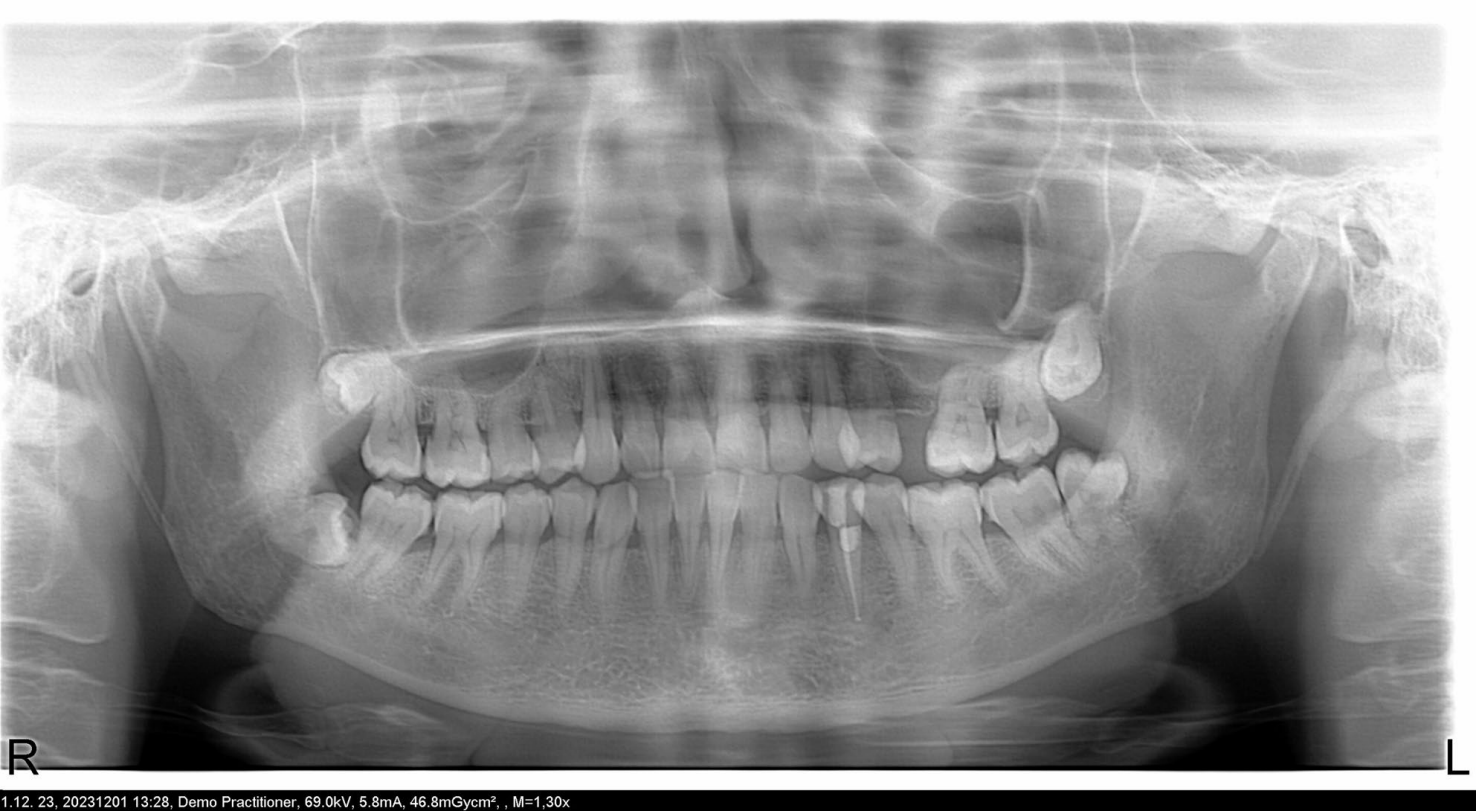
Panoramic radiograph of a systemically healthy individual showing pulp stones in the molar region
